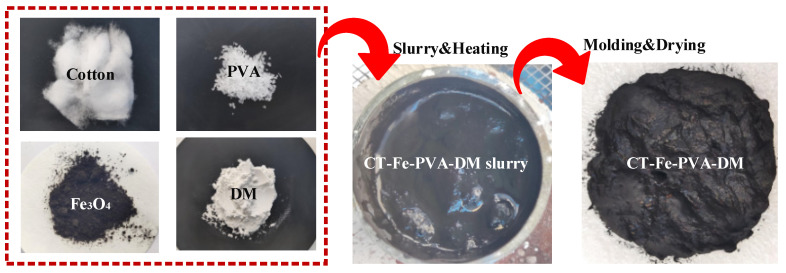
Preparation process of CT-Fe-PVA-DM.
